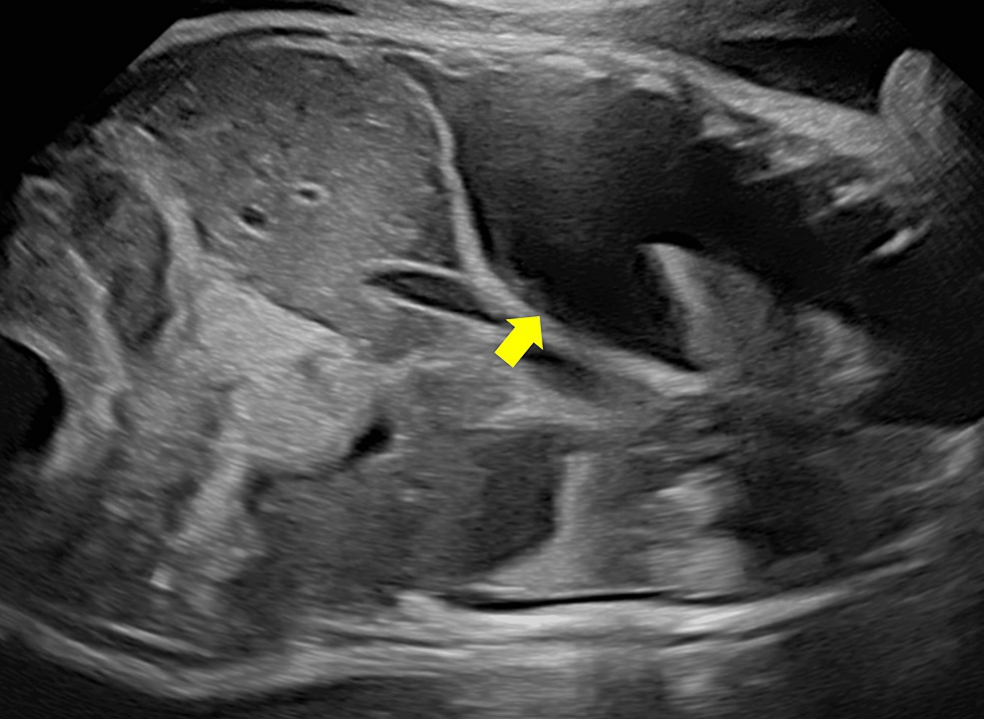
Fetal pleural effusion visualized on a fetal ultrasound image at 33 weeks, four days gestation (yellow arrow).
